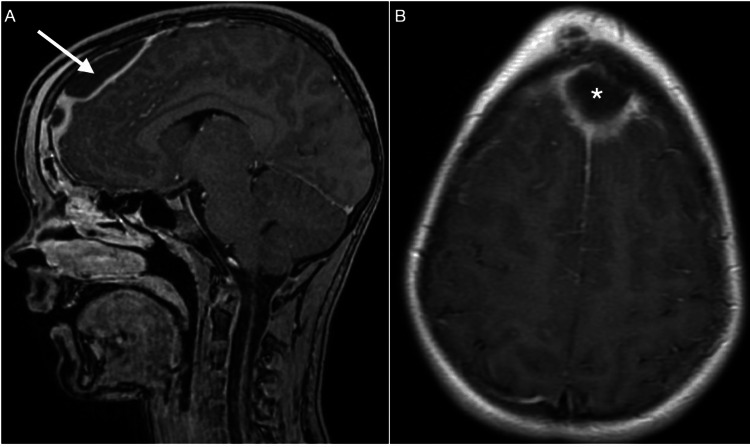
Preoperative MRI of Pott’s puffy tumor. Postgadolinium T1 MRI showing peripheral enhancement of the epidural collection exerting a mass effect on the frontal lobe in the sagittal slice (arrow) in (A), and proximity to the superior sagittal sinus is shown on the axial slice (asterisk) (B).
